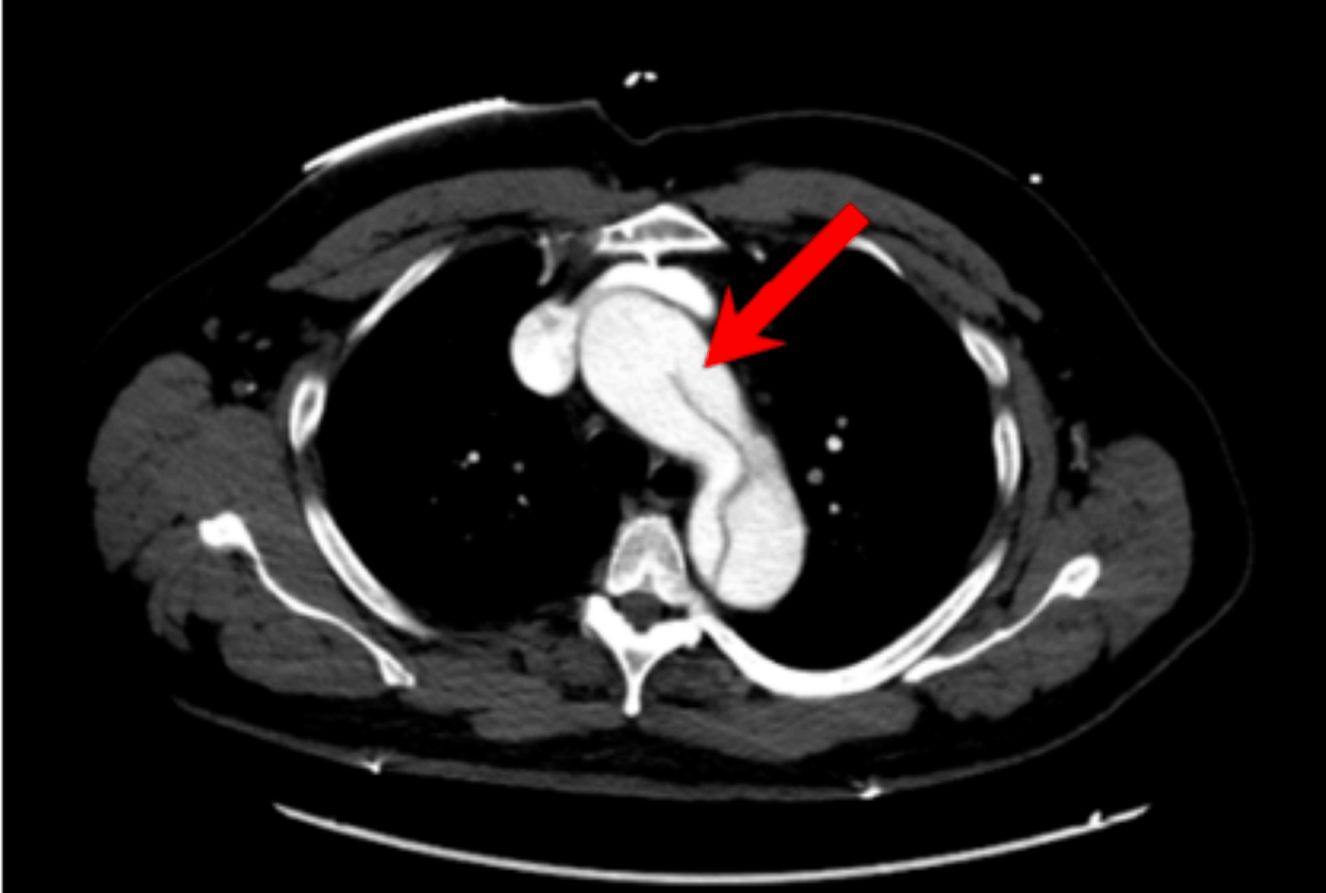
CTA chest axial view of aortic dissection (false lumen in aortic arch, red arrow) CTA: computed tomography angiography
